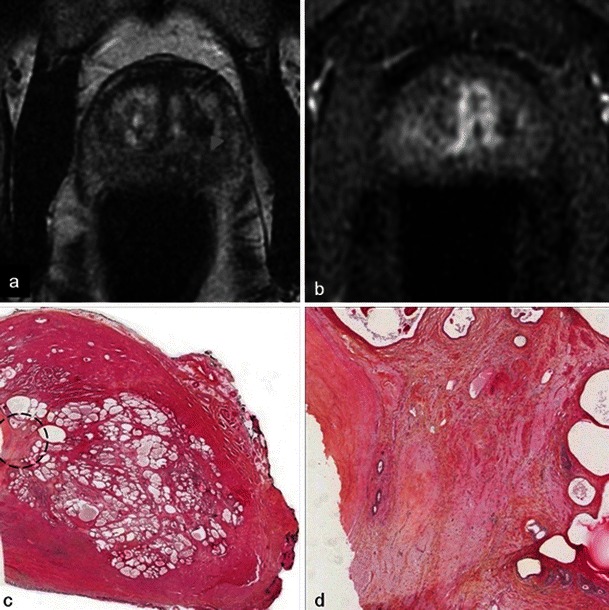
Fibrous scar. Axial T2-weighted MR (a) and axial T1 FS contrast-enhanced (b) images, in a case of bilateral photodynamic therapy show a post-treatment pattern, with a small area of low signal intensity, without enhancement, corresponding to fibrous scar tissue (*arrowhead*). Low power (c, d) images from radical prostatectomy show a focus of sclerotic and hyaline necrosis on the left lobe suggesting a 7 × 5-mm therapy scar
